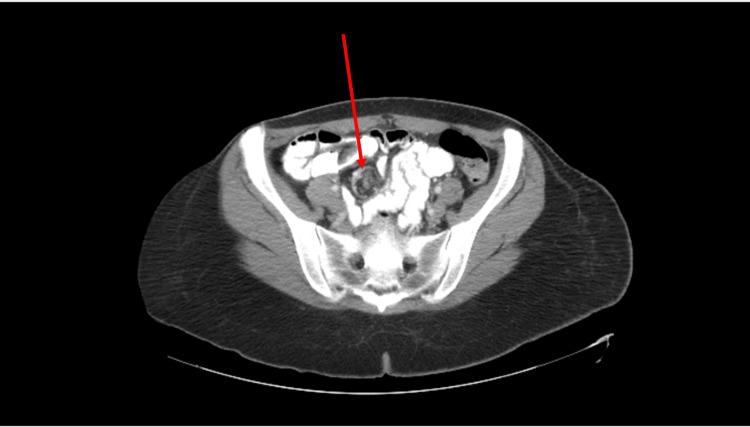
CT scan of the abdomen and pelvis with intravenous contrast showing an appendicular mass with a whirl sign. CT, computed tomography
